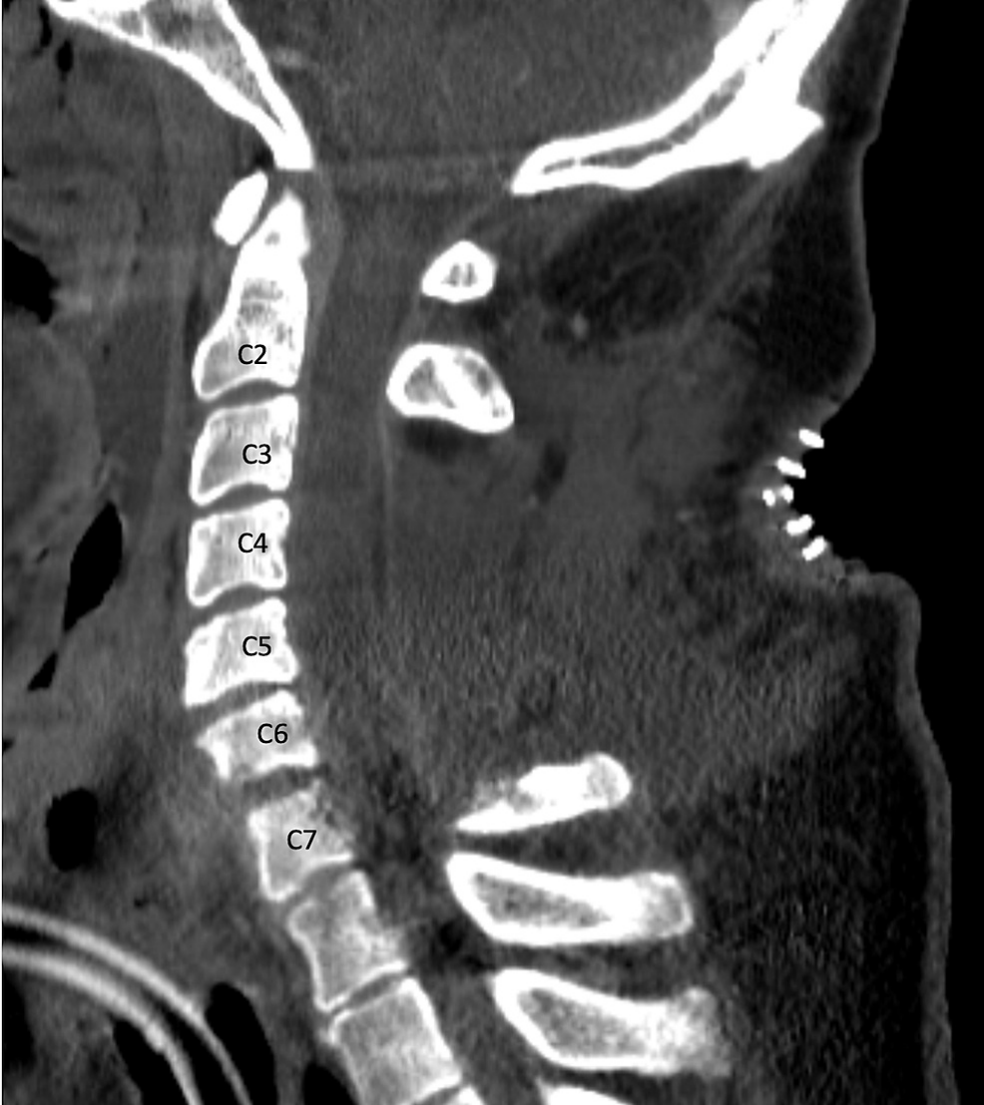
Postoperative Computed Tomography Scan Sagittal view of CT with contrast demonstrating postoperative changes after C3-C7 posterior laminectomy.
